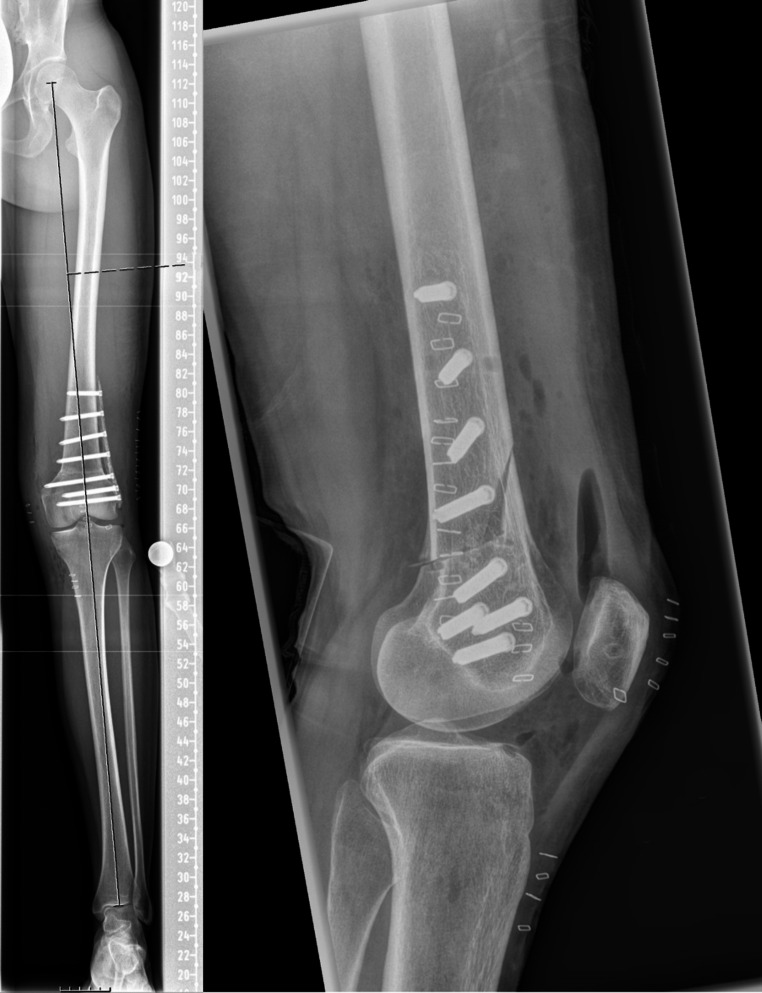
Postoperative whole-leg and lateral radiograph of concomitant derotational and varus-producing biplanar distal femoral osteotomy
